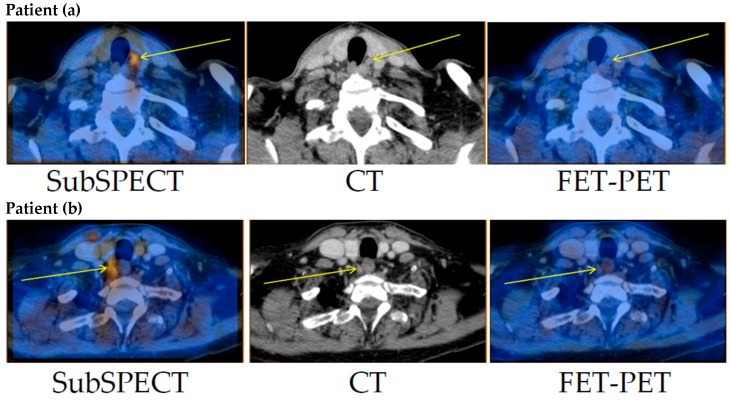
Tracer-uptake in the parathyroid adenomas in patients (a) and (b). Left: Subtraction (Tc-99m-sestamibi minus I-123)-SPECT/CT. Middle: CT. Right: FET-PET/CT. Thin arrows mark the surgically confirmed location of the parathyroid adenoma. FET: *O*-2-(^18^F)fluoroethyl-l-tyrosine; PET: positron emission tomography; SPECT/CT: single photon emission computed tomography/CT.
